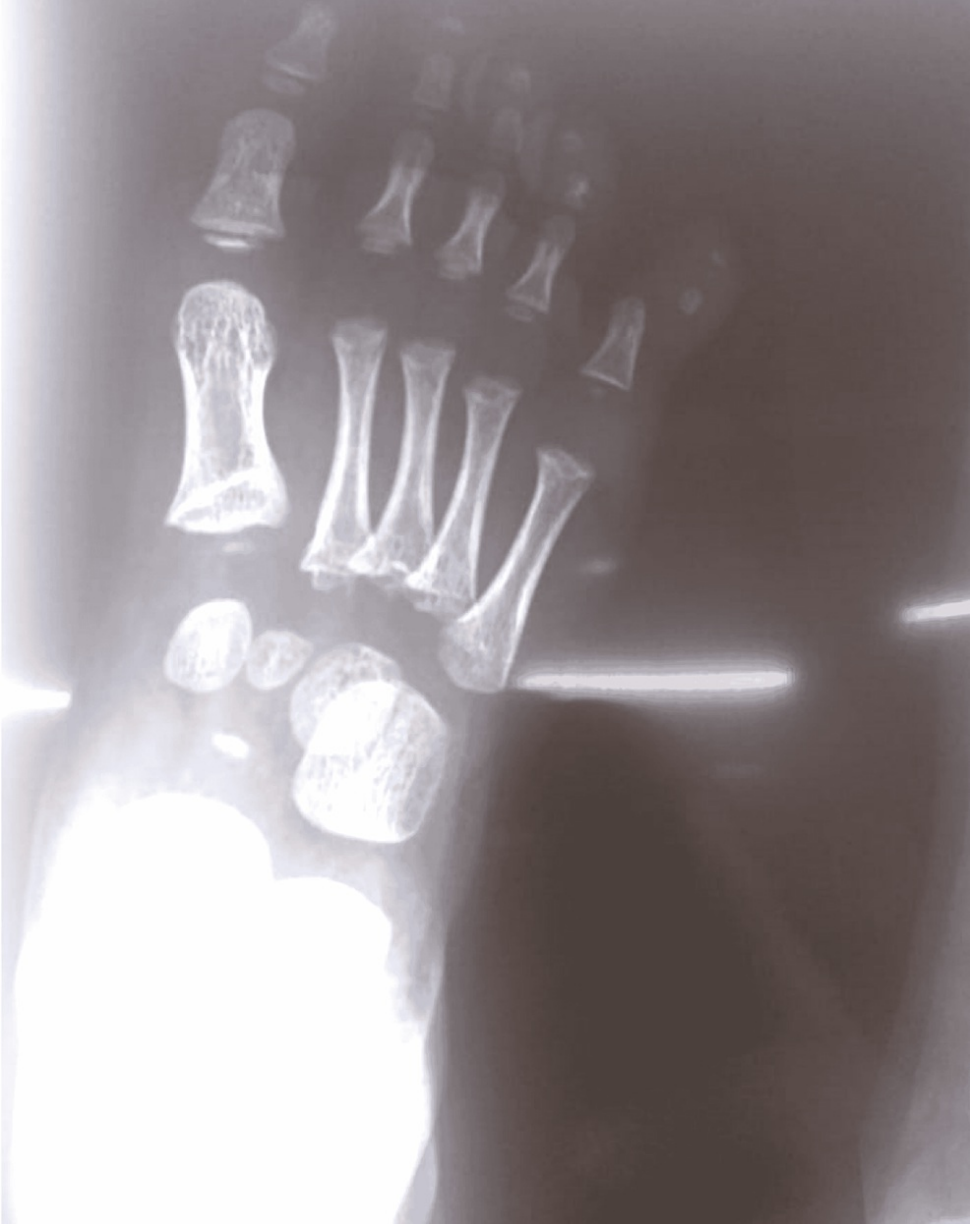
Radiograph on initial presentation Anteroposterior radiograph of the foot at initial presentation demonstrating a dorsolateral dislocation of the fifth digit proximal interphalangeal joint
